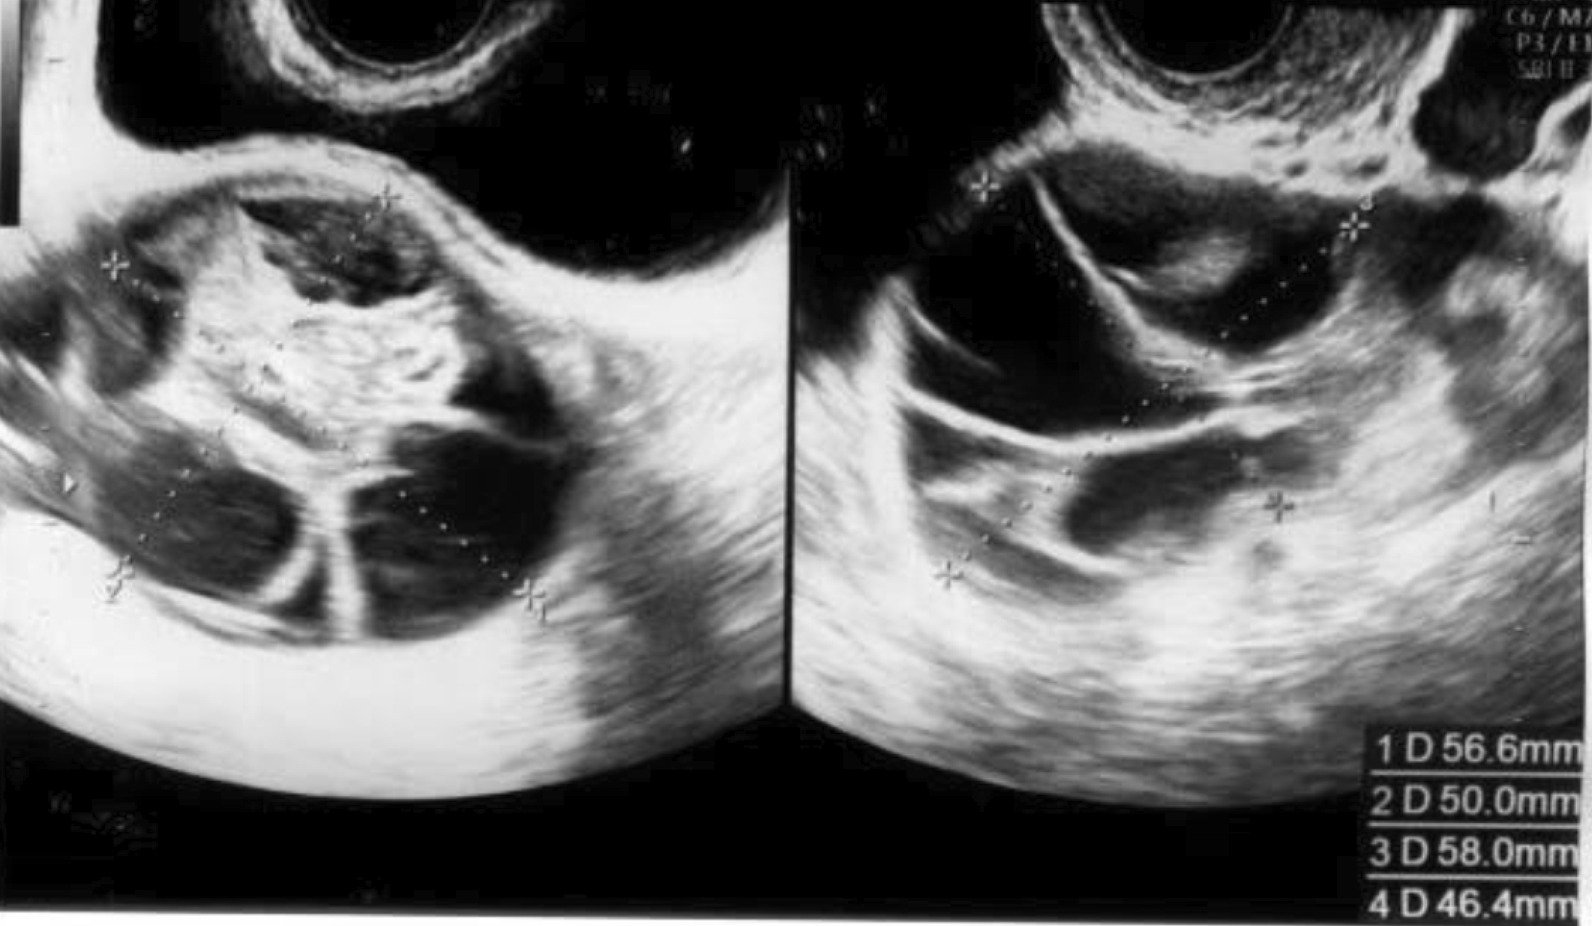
Ovarian ultrasonography showing mild ovarian swelling 2 days after oocyte retrieval
